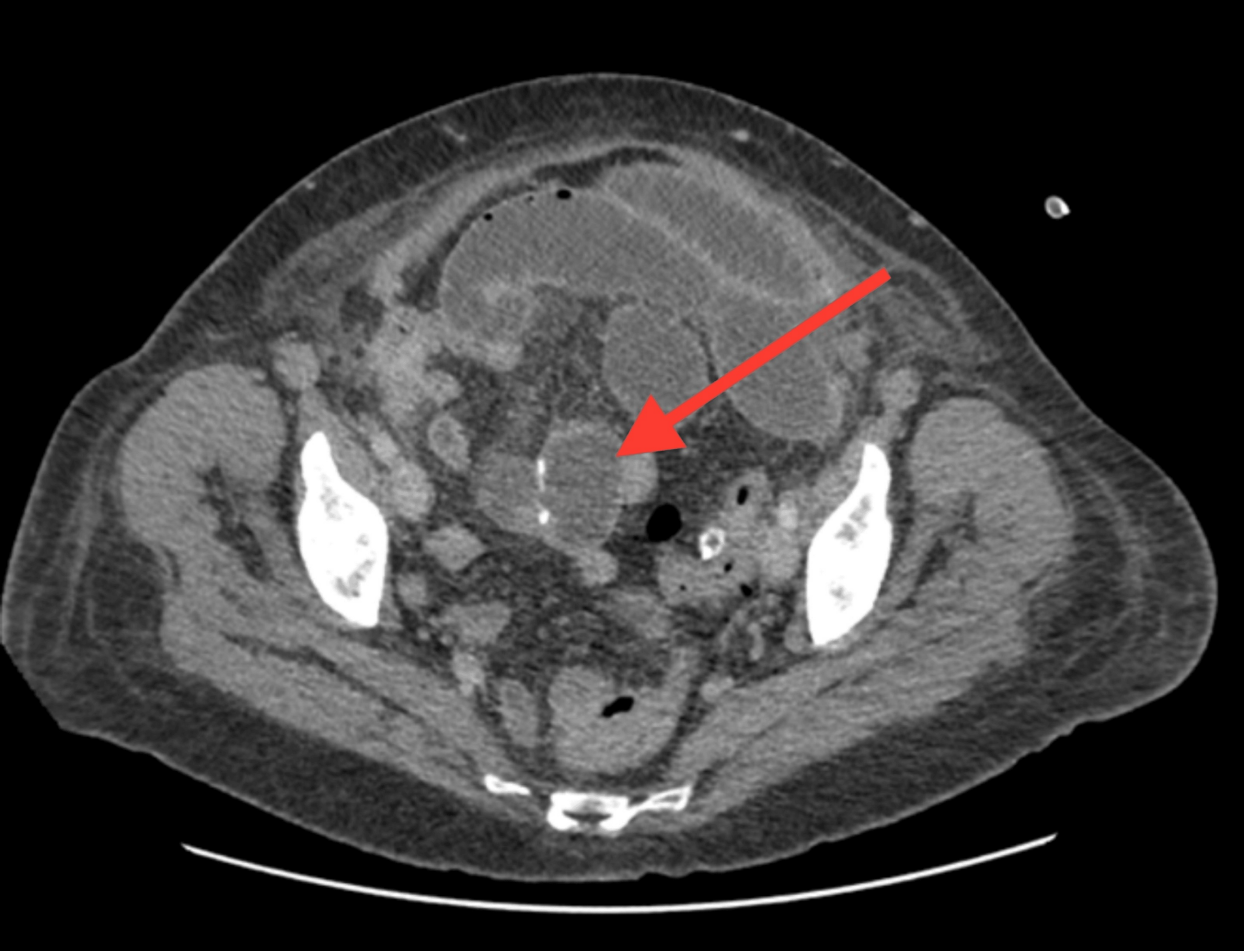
CT findings day 3 postoperative: dilated proximal loops, collapsed distal bowel, query transition point at the anastomotic site CT scan on postoperative day 3 showing markedly dilated proximal small bowel loops with collapsed distal loops. A possible transition point can be seen at the site of the small bowel anastomosis (suggesting anastomotic stricture)
